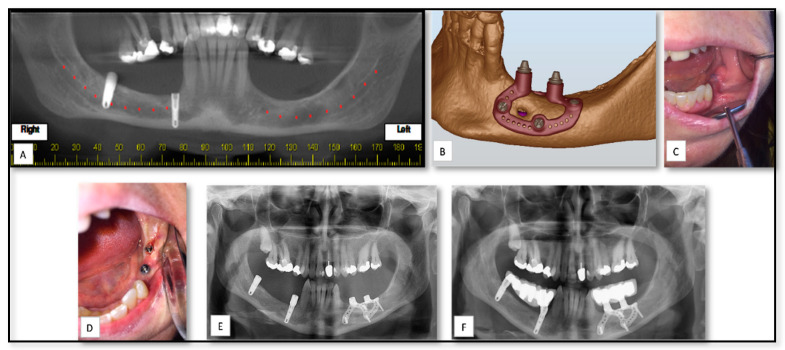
(A) Preoperative Cone beam computed tomography (CBCT) panoramic showed posterior edentulous mandible. (B) Pre-surgical planning and modeling of the sub-periosteal implant (C) One week after placement of the sub-periosteal implant shows proper healing. (D) One week after implant coverage and installation of dental healing caps. (E,F) Postoperative panoramic view showed the sub-periosteal implant with and without the final dental rehabilitation.
